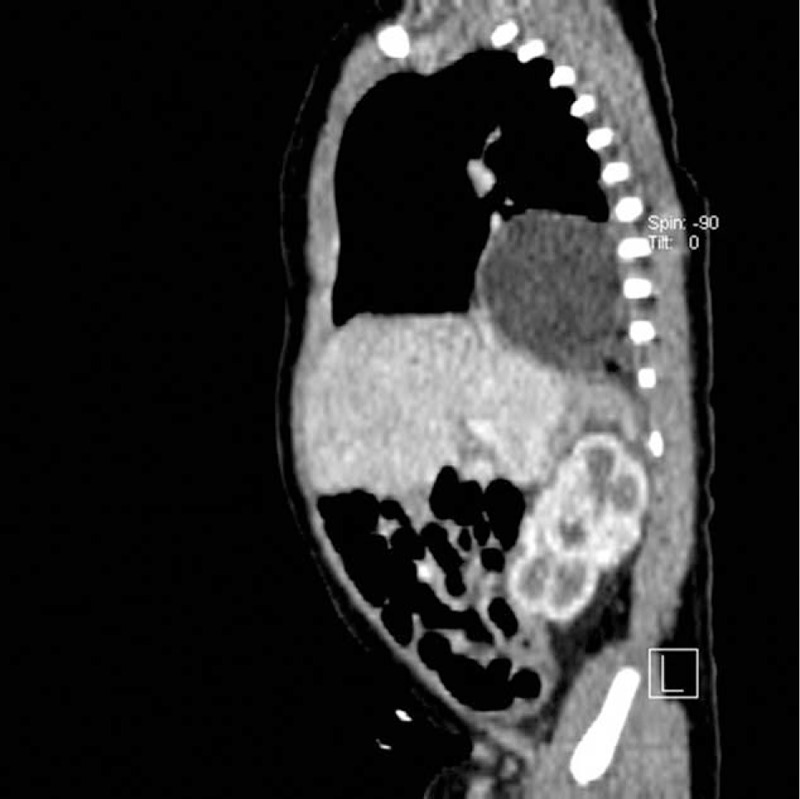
Computed tomography scan showed the well circumscribed mass in the posterior mediastinum (sagittal view).
